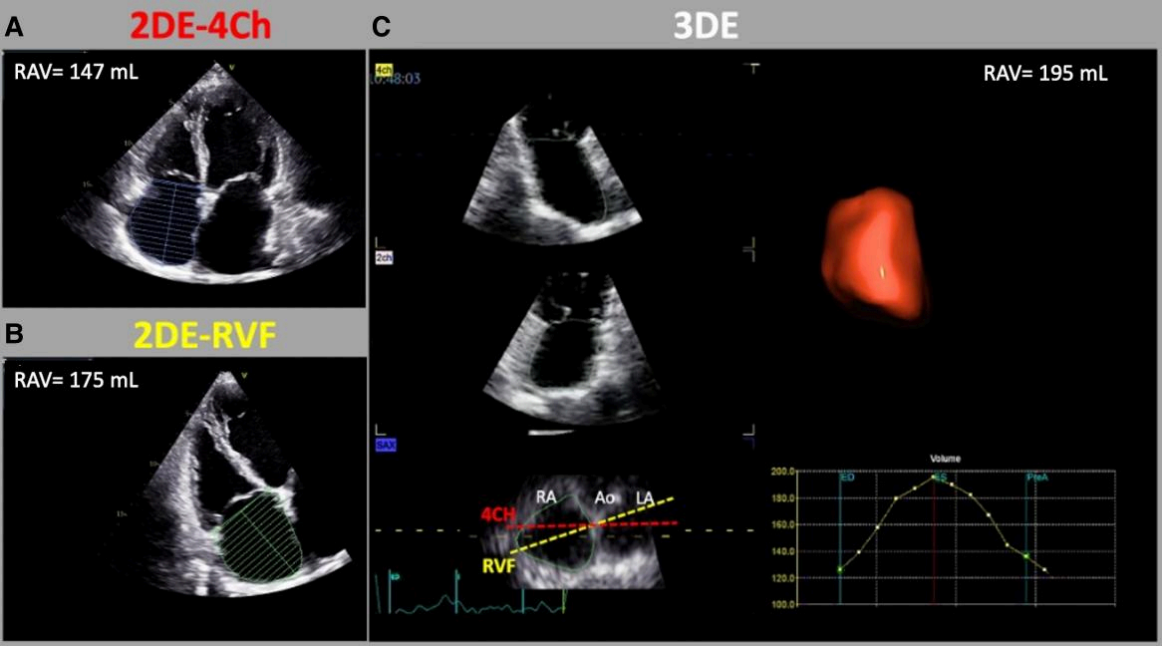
Measurement of RAV by 2DE using apical four-chamber view (*A*), apical right ventricular-focused view (*B*), and 3DE (*C*). 2DE, two-dimensional echocardiography; 3DE, three-dimensional echocardiography; 4Ch, four-chamber; Ao, aortic valve; LA, left atrium; RA, right atrium; RVF, right ventricular focused.
